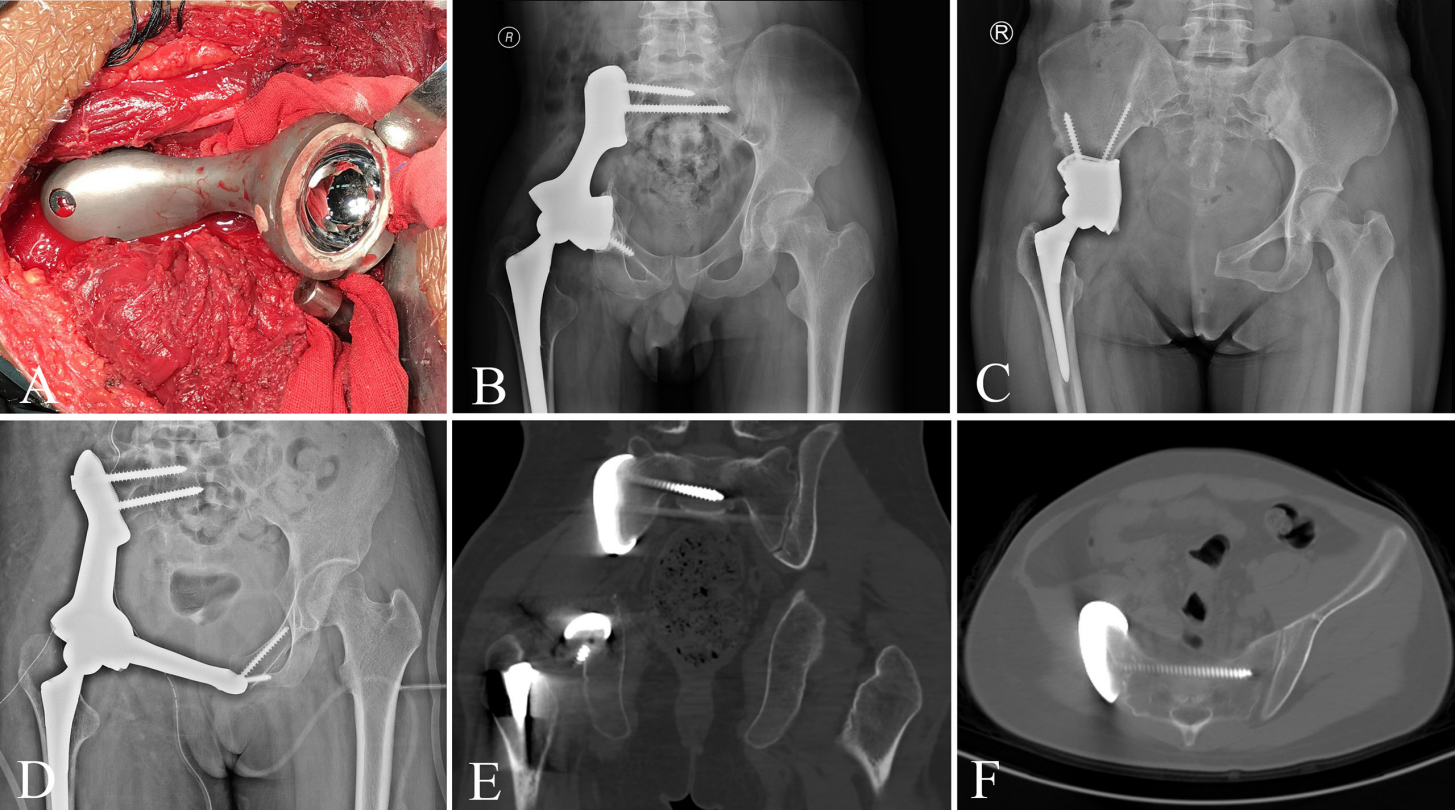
(A) The customized 3D-printed prosthesis was implanted following tumor resection. (B–D) Pelvic radiograph after 3D-printed prosthesis reconstruction. (E, F) CT scan shows that the bone is tightly bound to the prosthesis.
